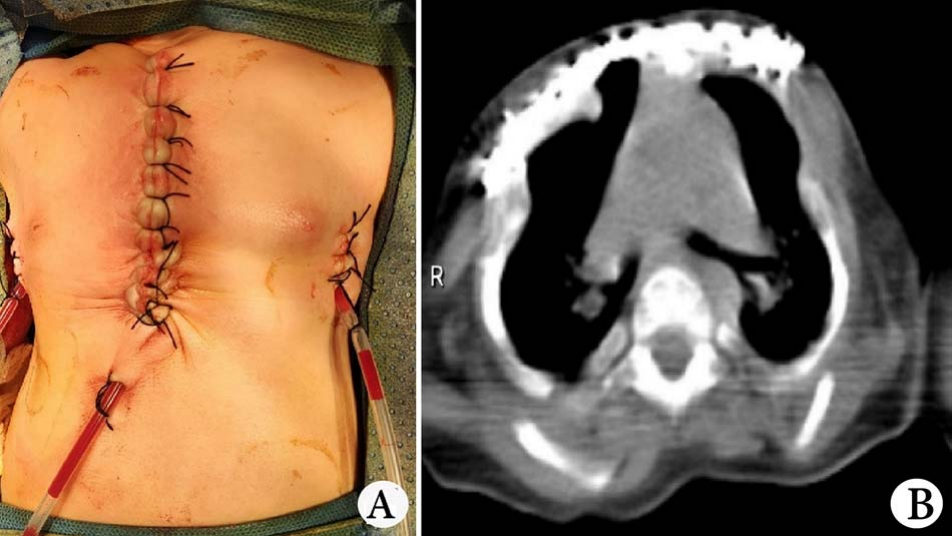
(A) Thorax appearance after operation, and (B) CT scan image of thorax after operation.
